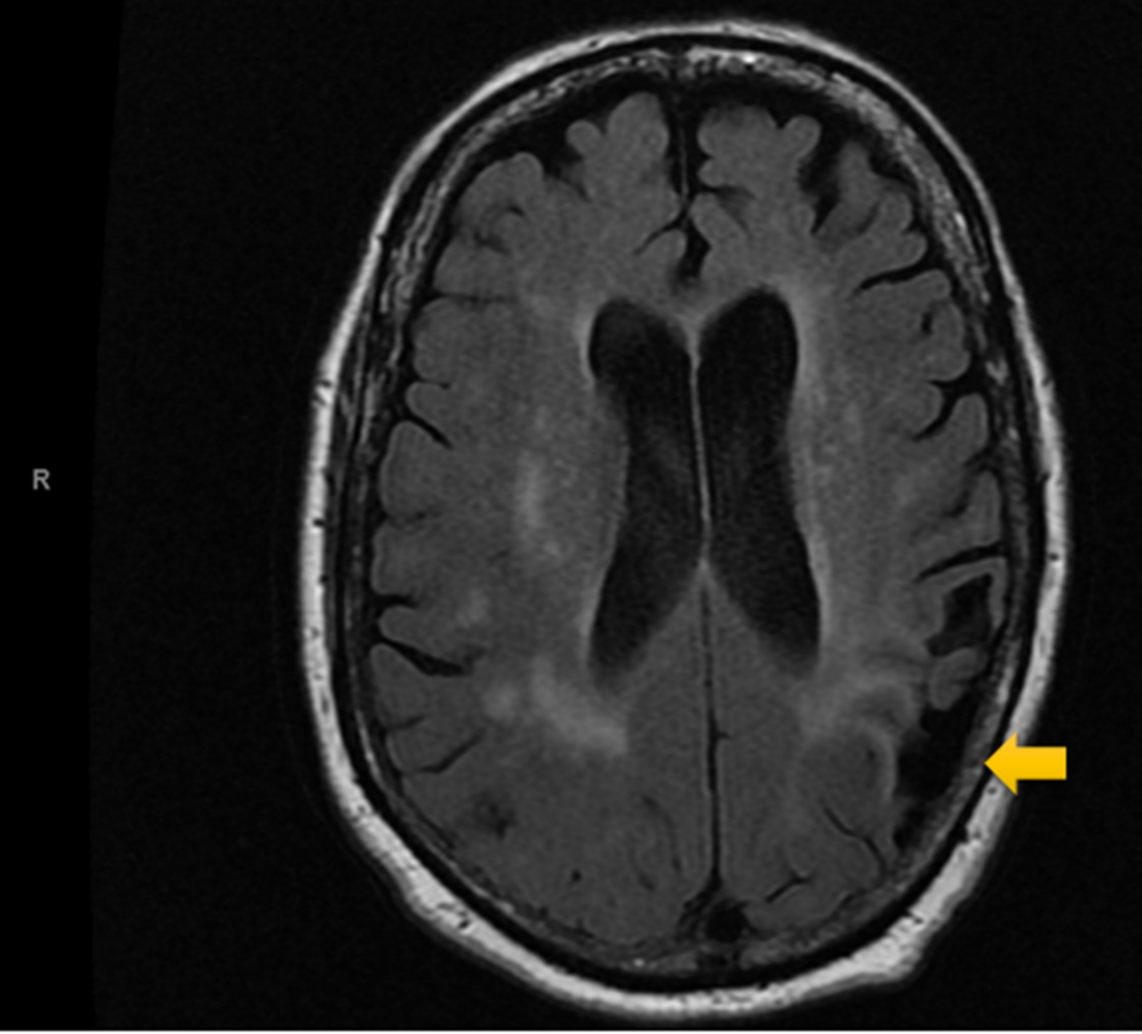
T2-flair MRI Scan of the Head Non-contrast T2-flair MRI showing diffuse cerebral atrophy along with point atrophy in the area of the suspected CVA in the parietal-temporal-occipital lobe. CVA, cerebrovascular accident.
